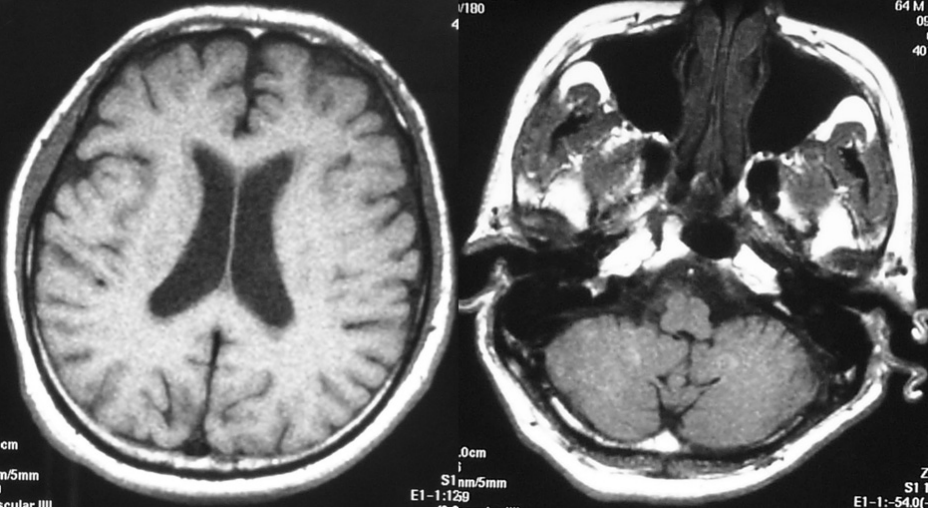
MRI of the brain showed no abnormal findings immediately after surgery.
